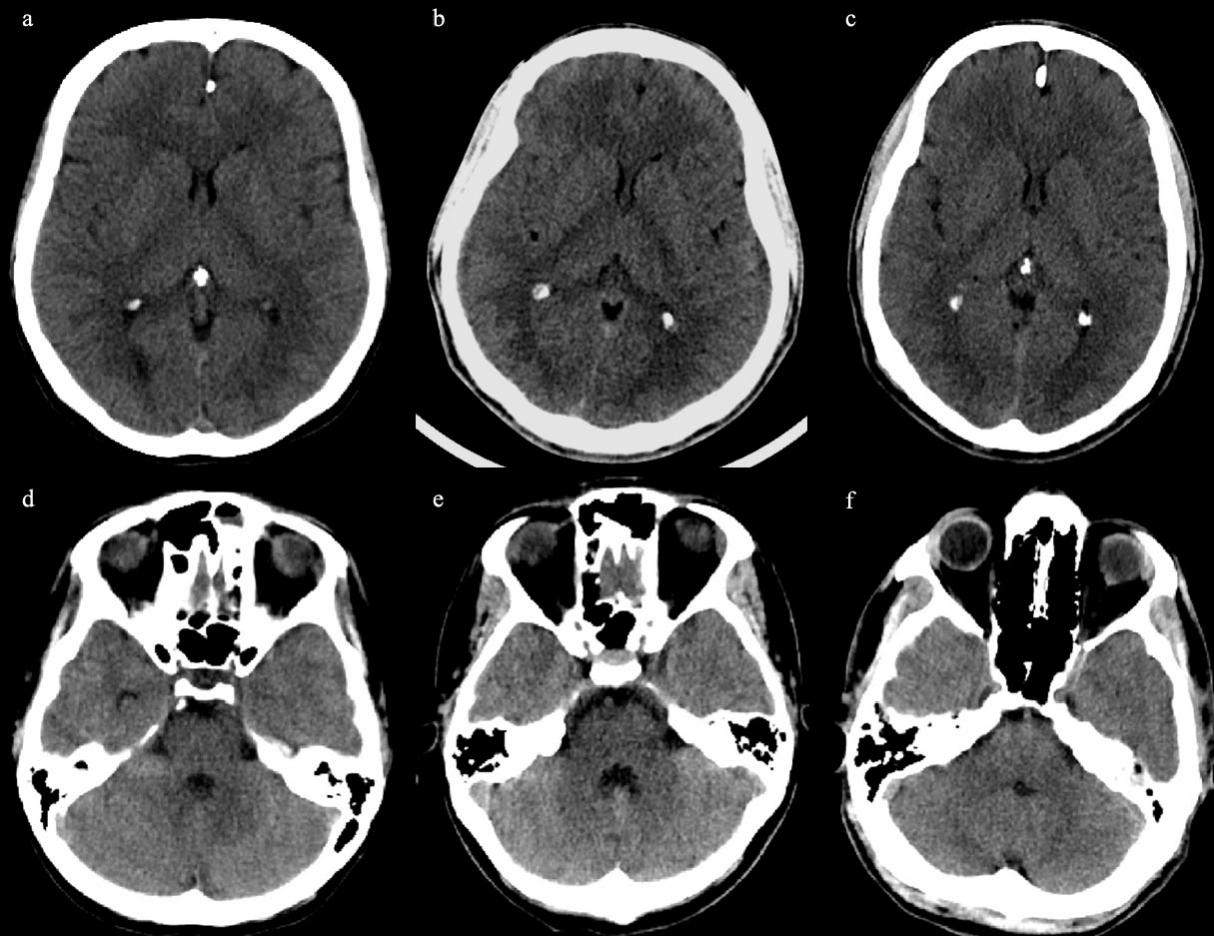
CT axial images of various protocols: (a) and (d) STD protocol (300 mAs, with FBP reconstruction); (b) and (e) LD‐I (250 mAs, with iDose^4^2); (c) and (f) LD‐II (200 mAs, iDose^4^3). The overall IQ is similar. FBP=filtered backprojection;IQ=image quality;LD=low dose;STD=standard dose.
